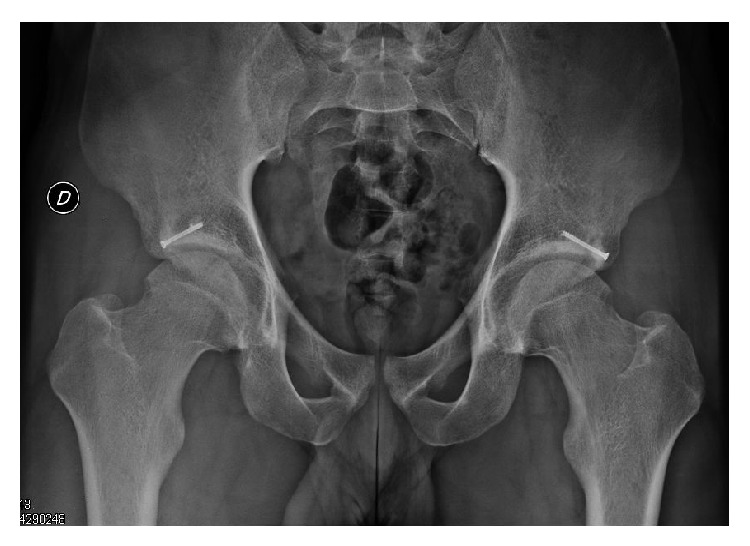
Pelvis AP radiographs at follow-up taken at* six months*  showed that the rim fracture healed. The LCE angle had decreased from 45° to 32° postoperatively in both hips and the hip joint space was still well maintained.
